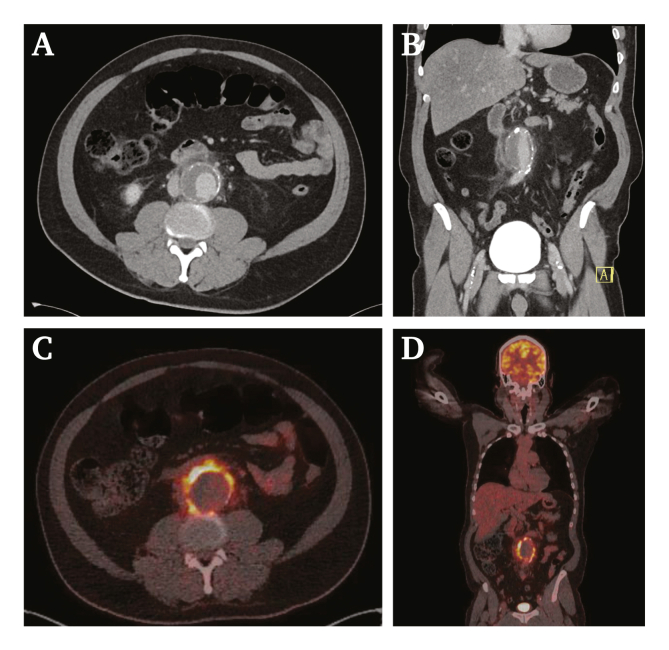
Computed tomography (CT) in axial (A) and coronal (B) view on admission demonstrating a 4.5 cm aortic aneurysm with inflammation of fatty tissue and fluid surrounding the aorta. ^18^F-fludeoxyglucose (FDG) positron emission tomography/low dose (ld) CT scan in axial (C) and coronal (D) view demonstrating significant heterogeneous FDG uptake of the aortic wall and an increase in diameter of the aortic aneurysm from 4.5 to 5.0 cm. FDG uptake was observed in retroperitoneal lymph nodes most likely reactive to the infected arterial wall. The spleen and bone marrow also showed weak uptake suggestive of an active infection. Physiological FDG uptake was seen in the brain and myocardium with signs of renal tracer excretion.
